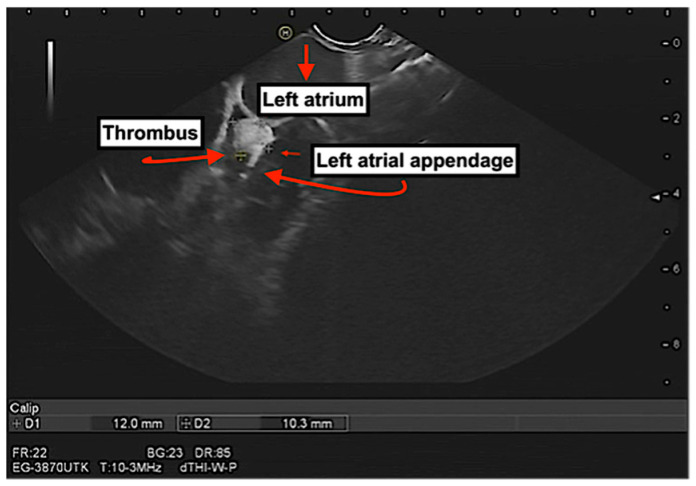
Endoscopic ultrasound demonstrated the morphology and thrombus in left atrial appendage.
